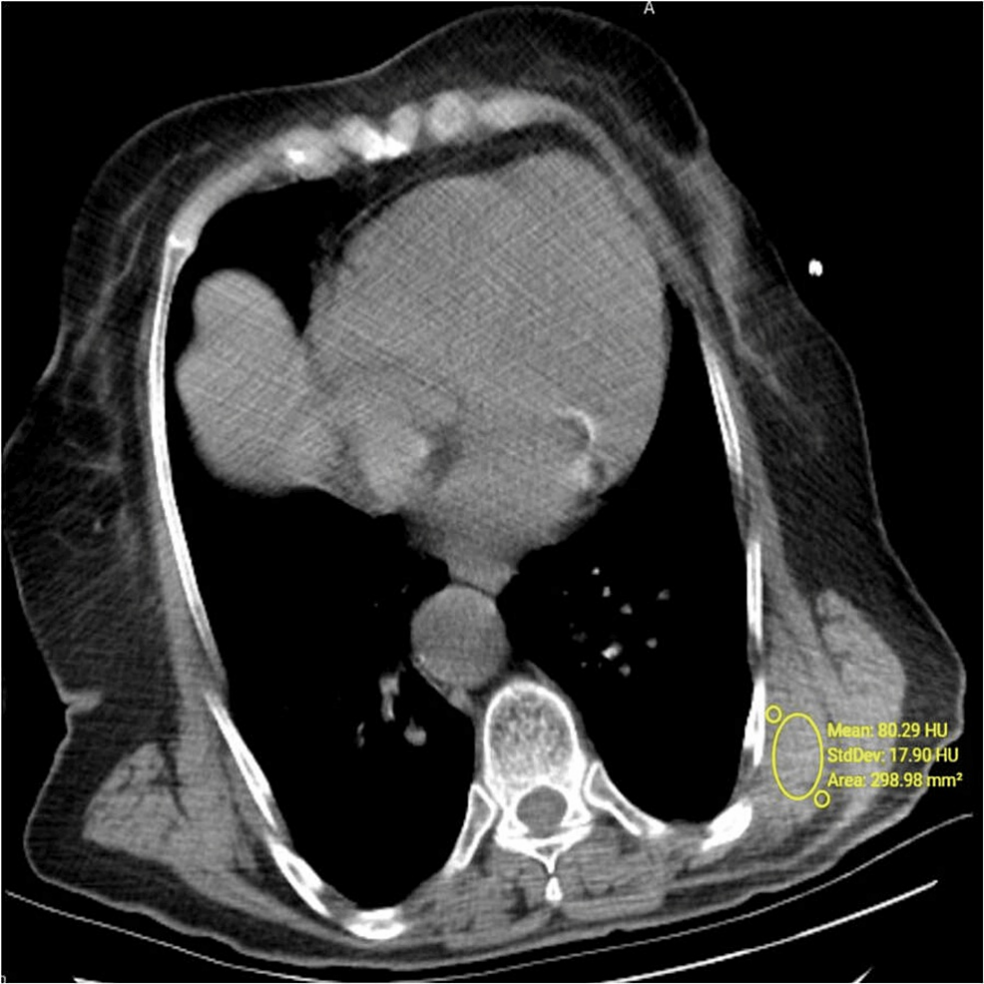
Axial non-contrast CT image. Density was measured to be +80 HU for left-side ED in a 78-year-old female patient.
